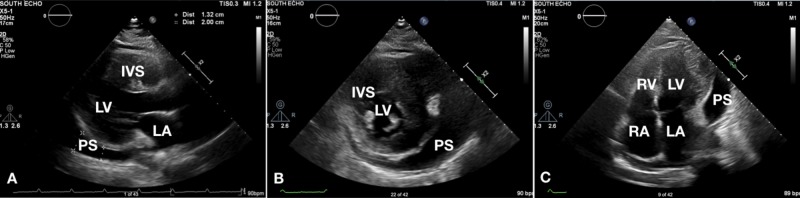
Representative echocardiographic images Parasternal long (A), parasternal short (B), and apical four-chamber views demonstrate pericardial effusion, thickened left ventricle wall, and interventricular septum LA: left atrium, LV: left ventricle, RA: right atrium, RV: right ventricle, IVS: interventricular septum, PS: pericardial effusion
